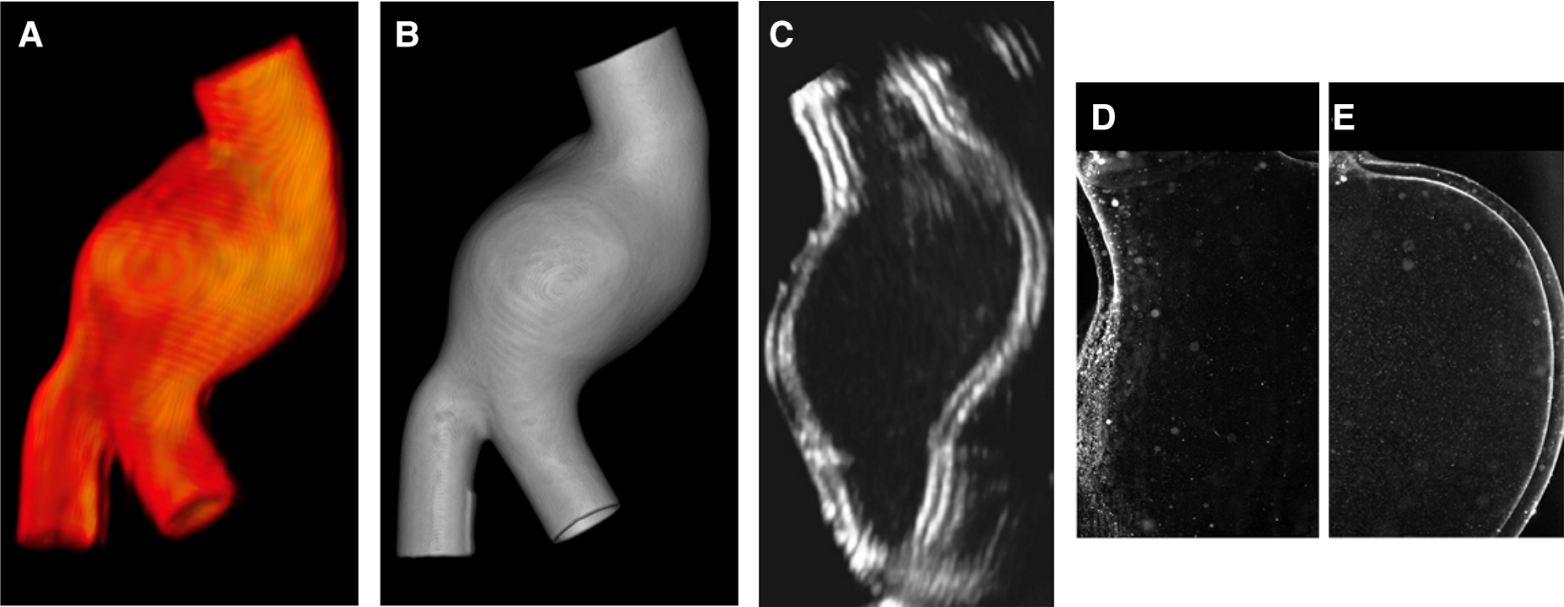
Render of MRI (A) and CT (B) scans acquired on LMM; B-mode Doppler acquisition in longitudinal view (C); PIV images (D–E).
